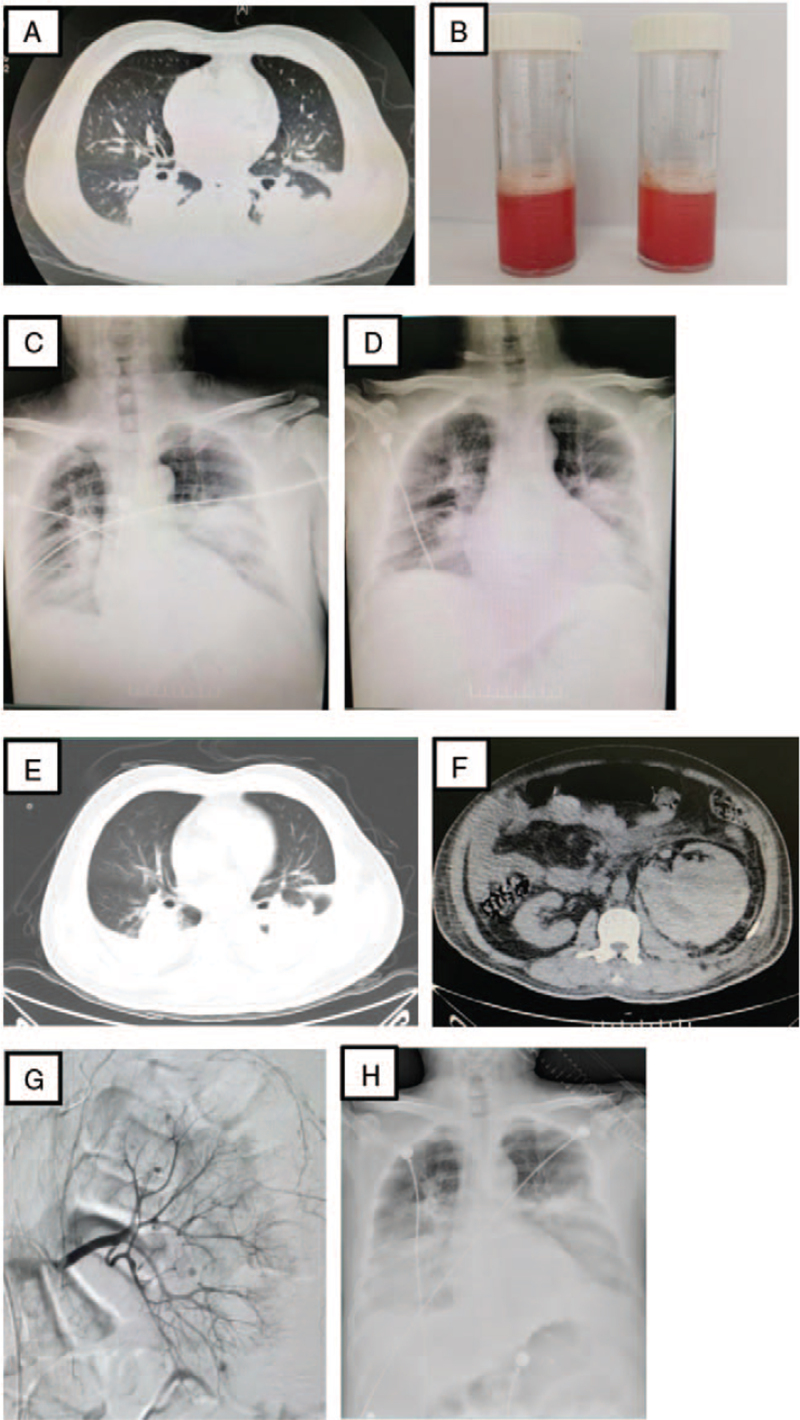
Imaging examinations during hospitalization. (A) On admission, chest X-ray showed bilateral infiltrates in the lower lobe of the lung. (B) Hemorrhagic bronchoalveolar lavage fluid by fiberoptic bronchoscopy. (C) On day 3 of admission, increased lung infiltrates were seen on chest X-ray. (D) On day 6 of admission, bilateral infiltrates in the lung were improved. (E) On day 10 of admission, increased bilateral infiltrates in the lower lobe of the lung were seen on the chest CT scan. (F) Abdominal CT scan showed left kidney and left retroperitoneal hematoma. (G) Selective arterial angiography showed multiple aneurysms in renal arteries. CT = computed tomography.
